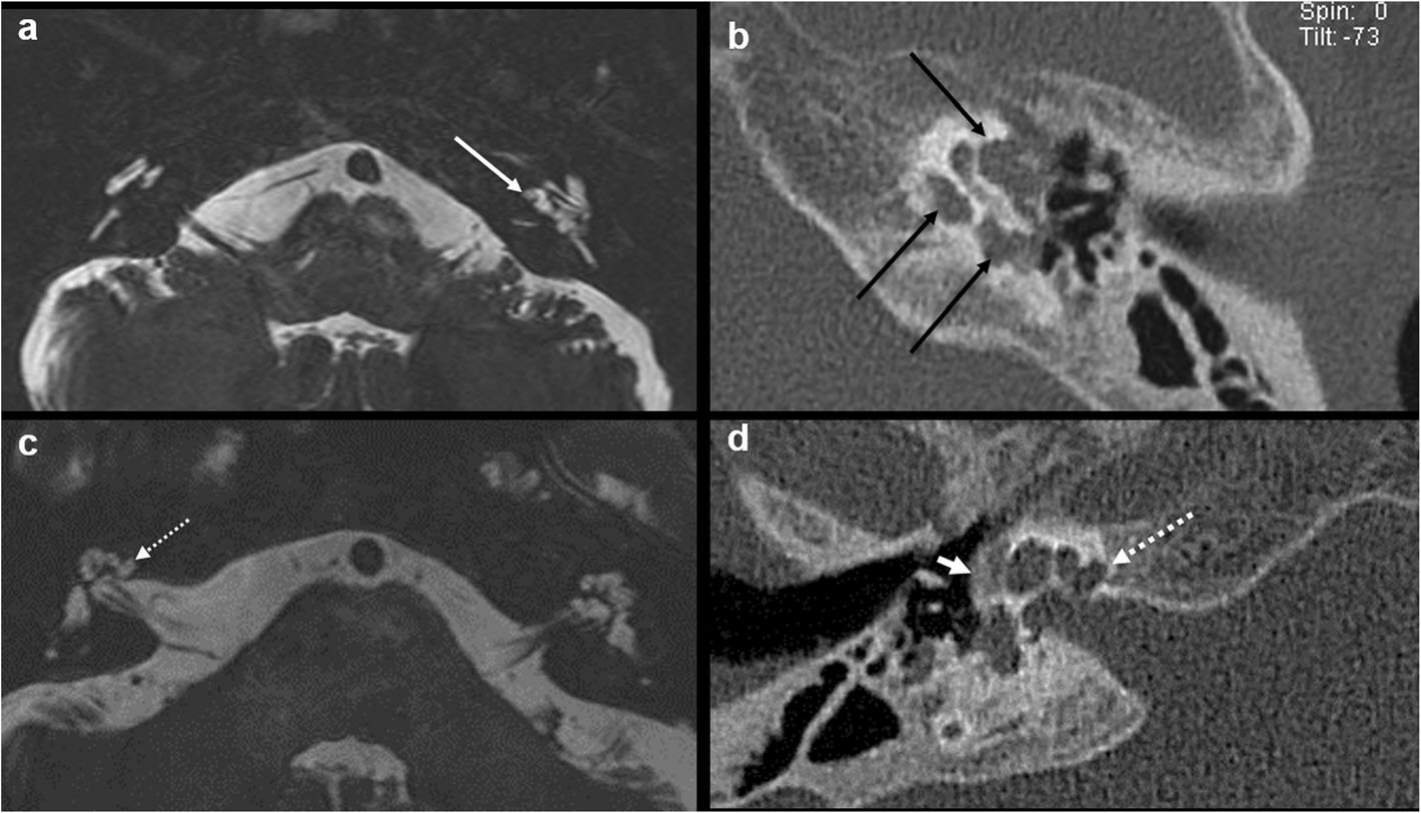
A 64-year-old male patient with heavy bilateral SNHL (case 7). Axial CISS image (a) shows curvilinear T2 hyperintensities (arrow) surrounding the left cochlea. Subsequent axial HRCT image (b) confirms extensive very hypodense plaques (arrows) in the left pericochlear region, likely long-standing disease. Axial CISS image (c) at a lower level also shows a small right IAC diverticulum (dashed arrow), confirmed on subsequent axial HRCT image (d) as an ‘ear-like’ outpouching anterior to the right IAC (dashed arrow). Also note the large plaque in the right FA region (short arrow)
